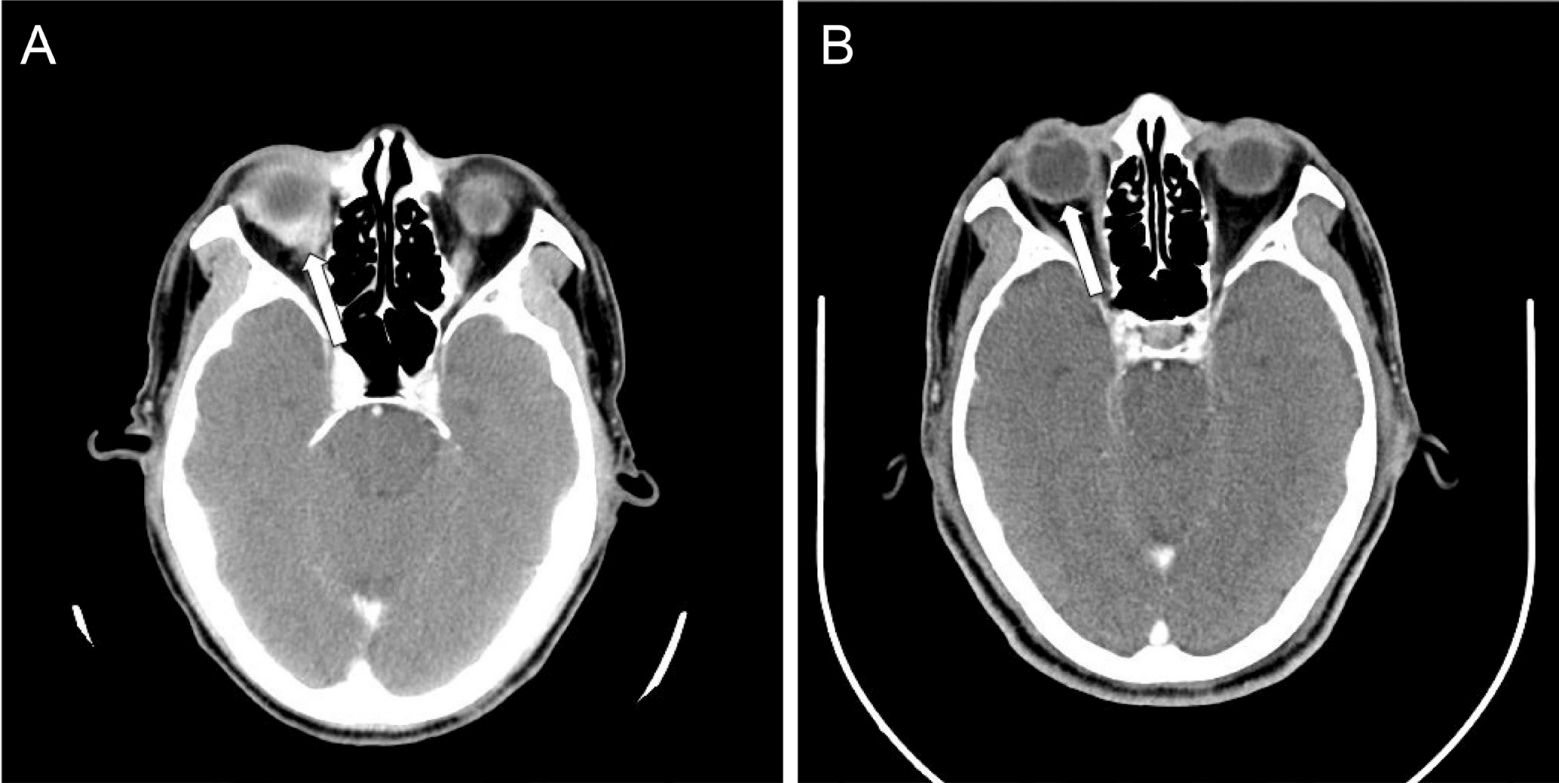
The CT scan of the brain with contrast showing (A) before treatment, the enhanced soft tissue infiltrates the right eyeball (arrow); and (B) after four cycles of R-CHOP, the tissue resolved with CR.
